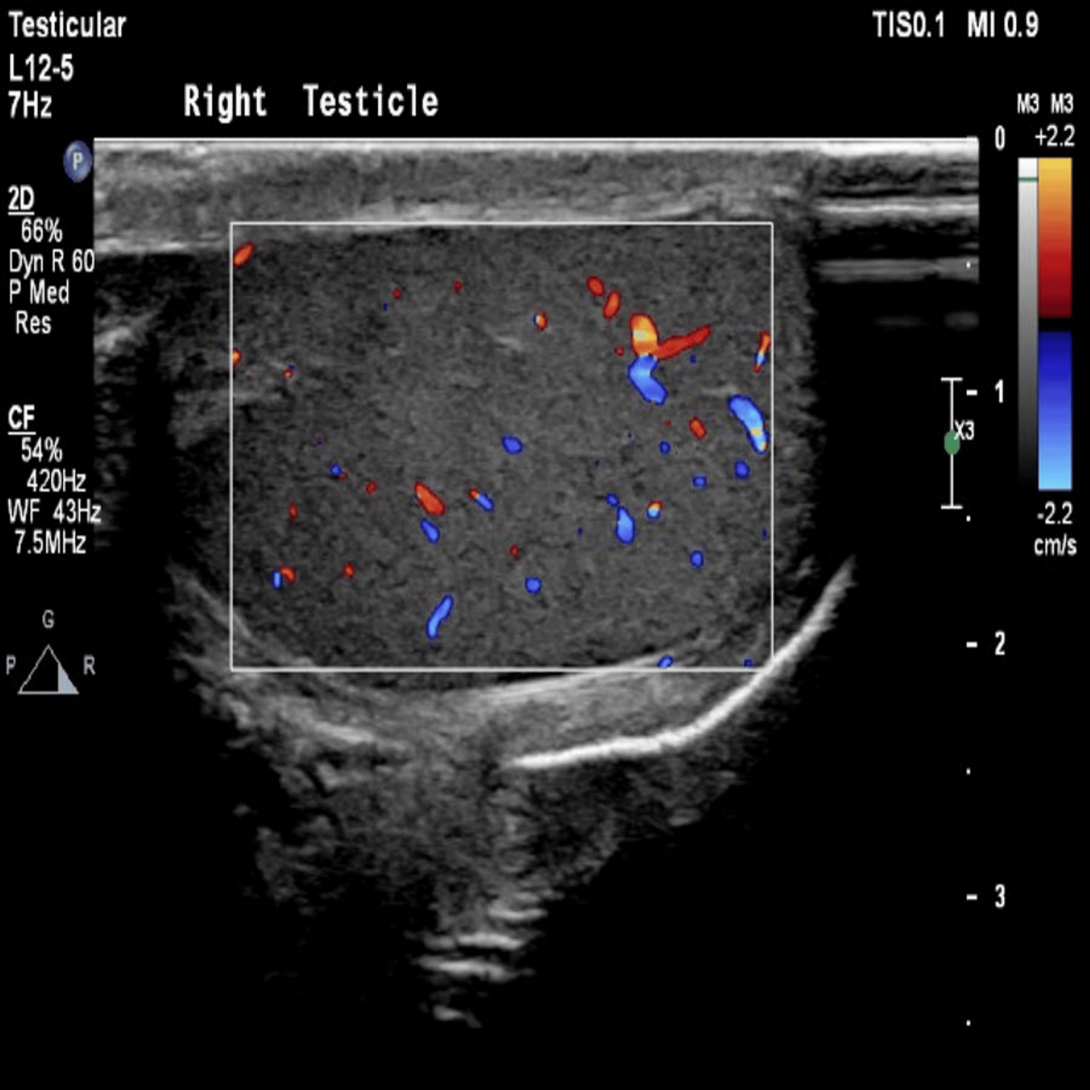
Ultrasound image of right testicle demonstrating homogeneous echogenicity with preserved vascularity
